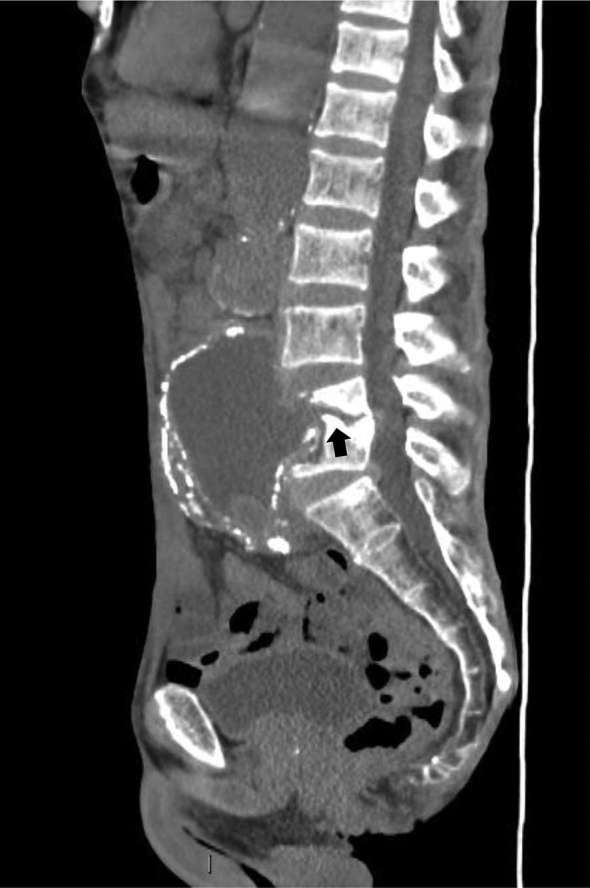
Sagittal CT showing communication of aneurysmal sac with L4/5 disc (arrow) with endplate destruction.
